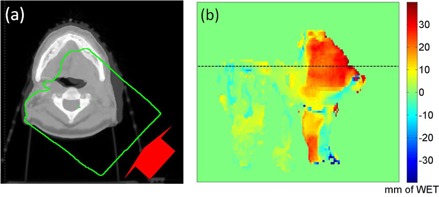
Axial slice (a) indicating the region of PDR calculation (green mask) from the proton source up to the distal 90% dose falloff; (b) beam's eye view PDR difference between the pCT and the cCBCT. The horizontal line indicates the corresponding CT slice in (a). Color scale bar is in mm.
